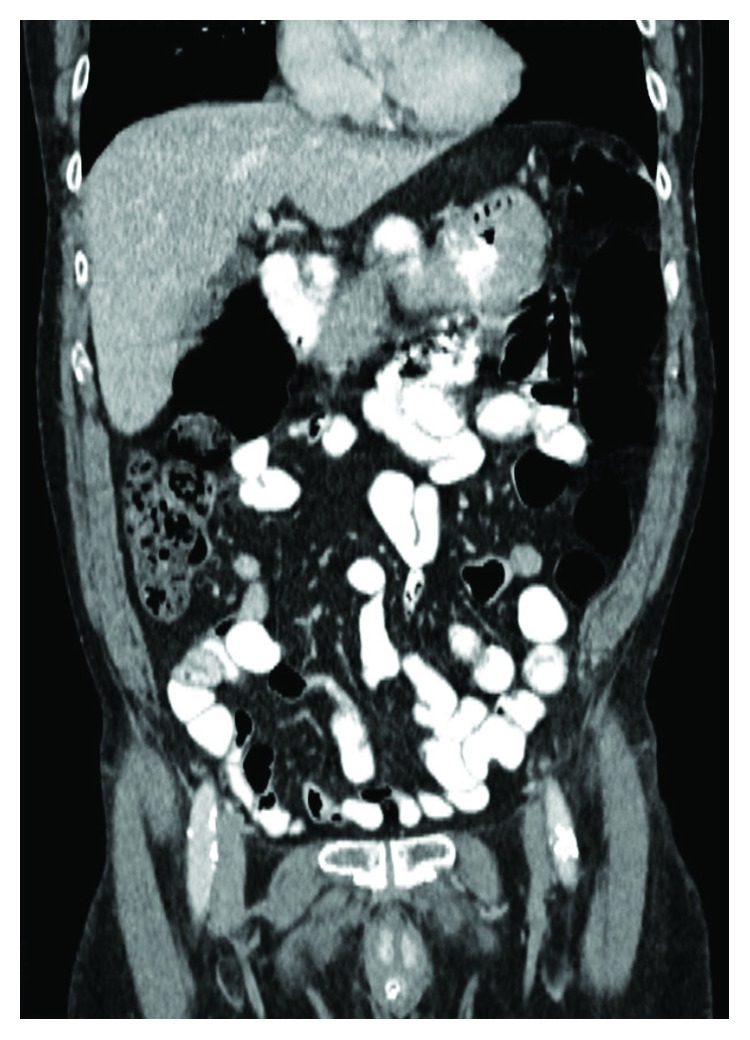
Coronary thoracoabdominal CT scan on admission without diaphragmatic hernia.
